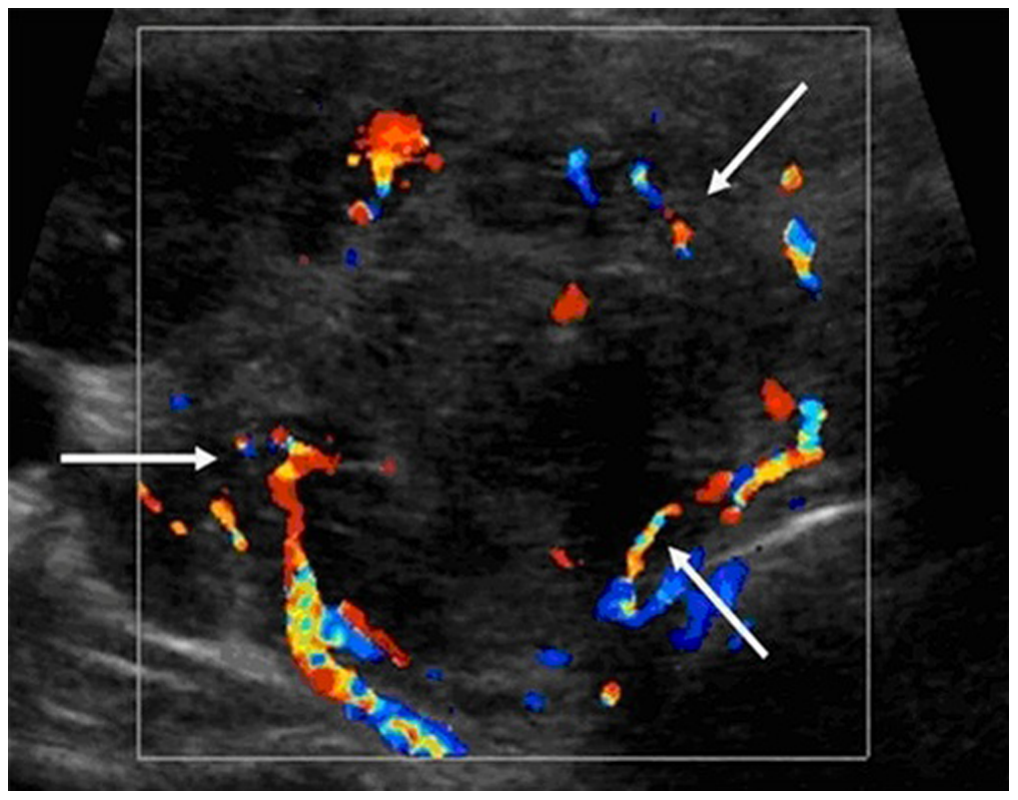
A 56-year-old female patient with non-diffuse PTL. The transverse sonogram shows the increased chaotic vascularity (arrows). (Tr: Trachea)
